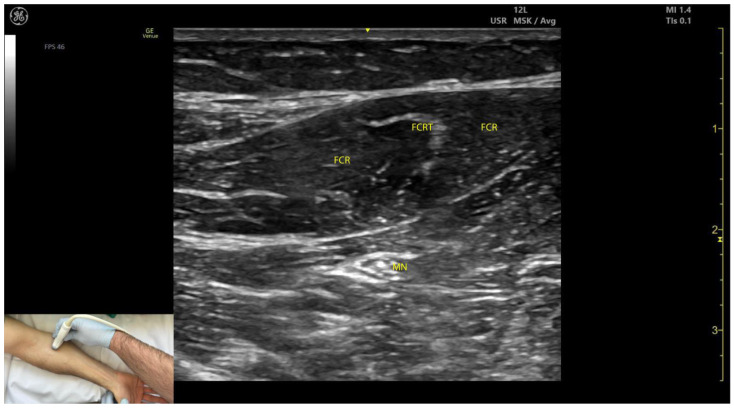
Ultrasound anatomy of the flexor carpi radialis (FCR) with key landmarks: FCR—flexor carpi radialis; FCRT—flexor carpi radialis tendon; MN—median nerve.
